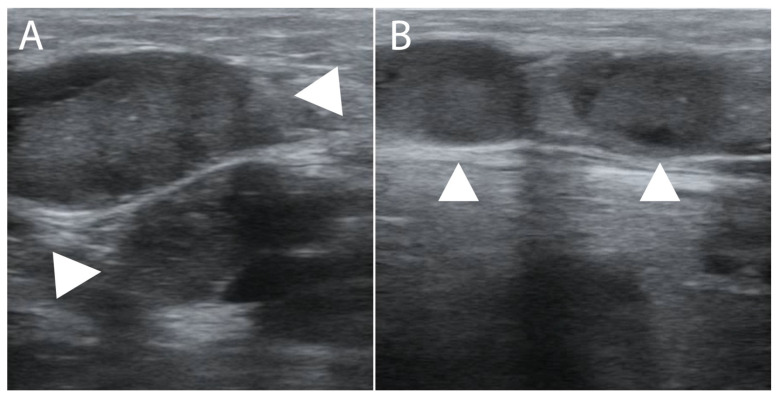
Multiple enlarged inguinal lymph nodes (arrowheads) were identified by ultrasound (A,B).
